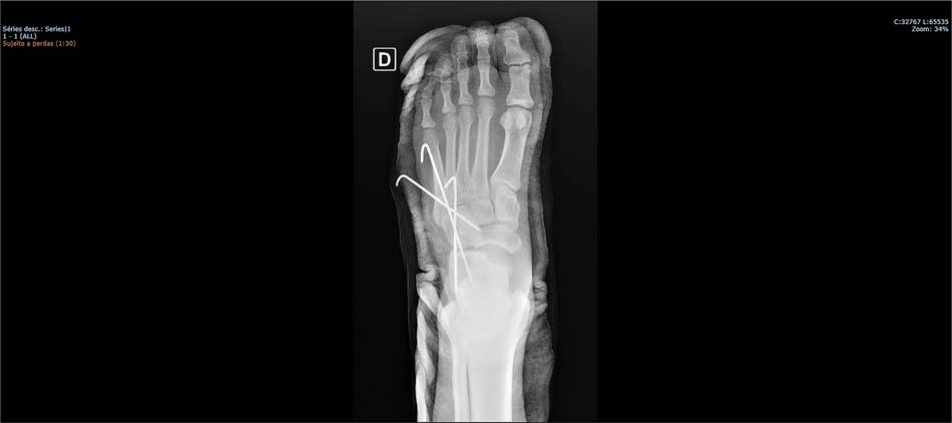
Postoperative X-ray of the foot in AP view
